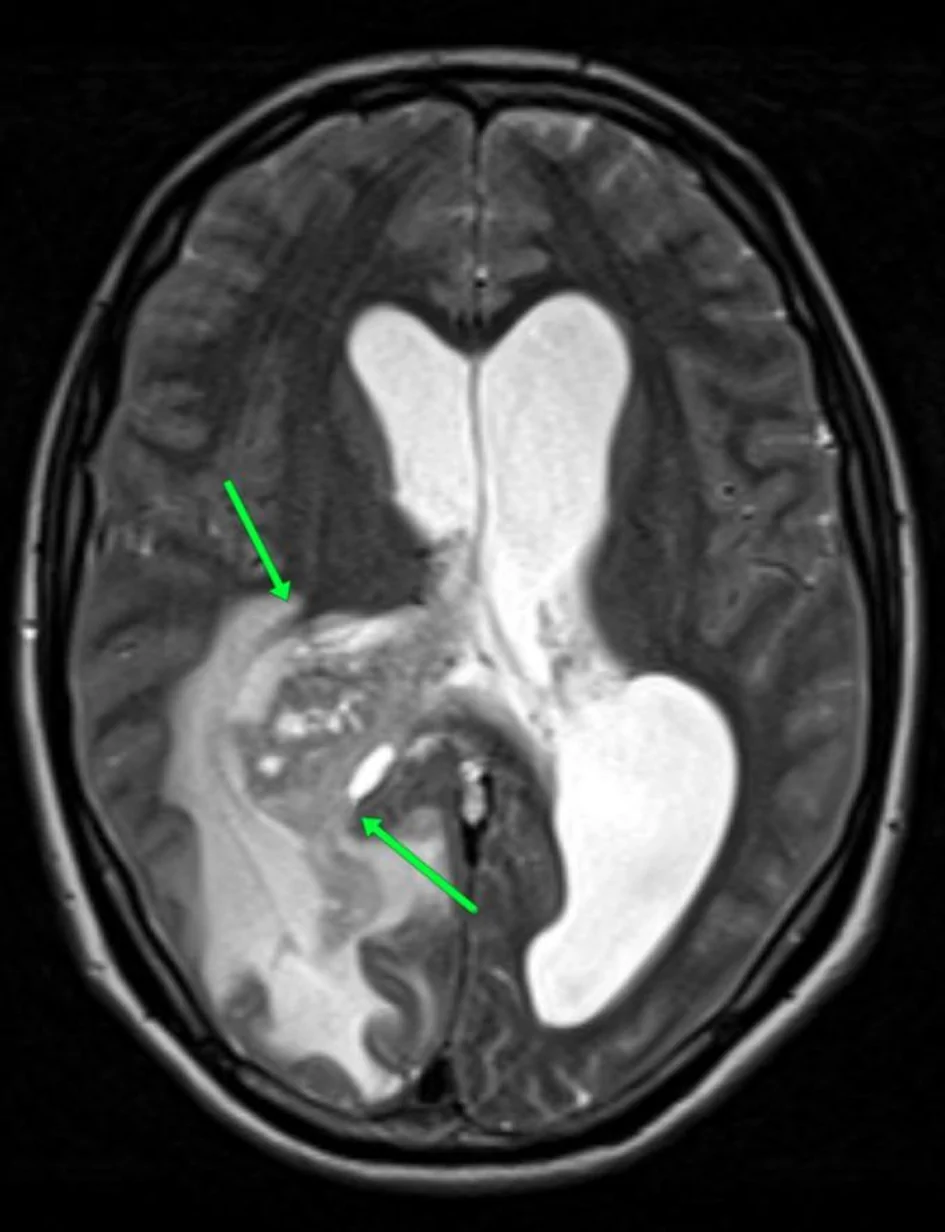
Axial T2-weighted MRI demonstrating a large intraventricular mass (green arrows) arising from the choroid plexus in the atrium of the right lateral ventricle, measuring approximately 6–7 cm in maximal dimension and greater than 3 cm in thickness. MRI: magnetic resonance imaging.
